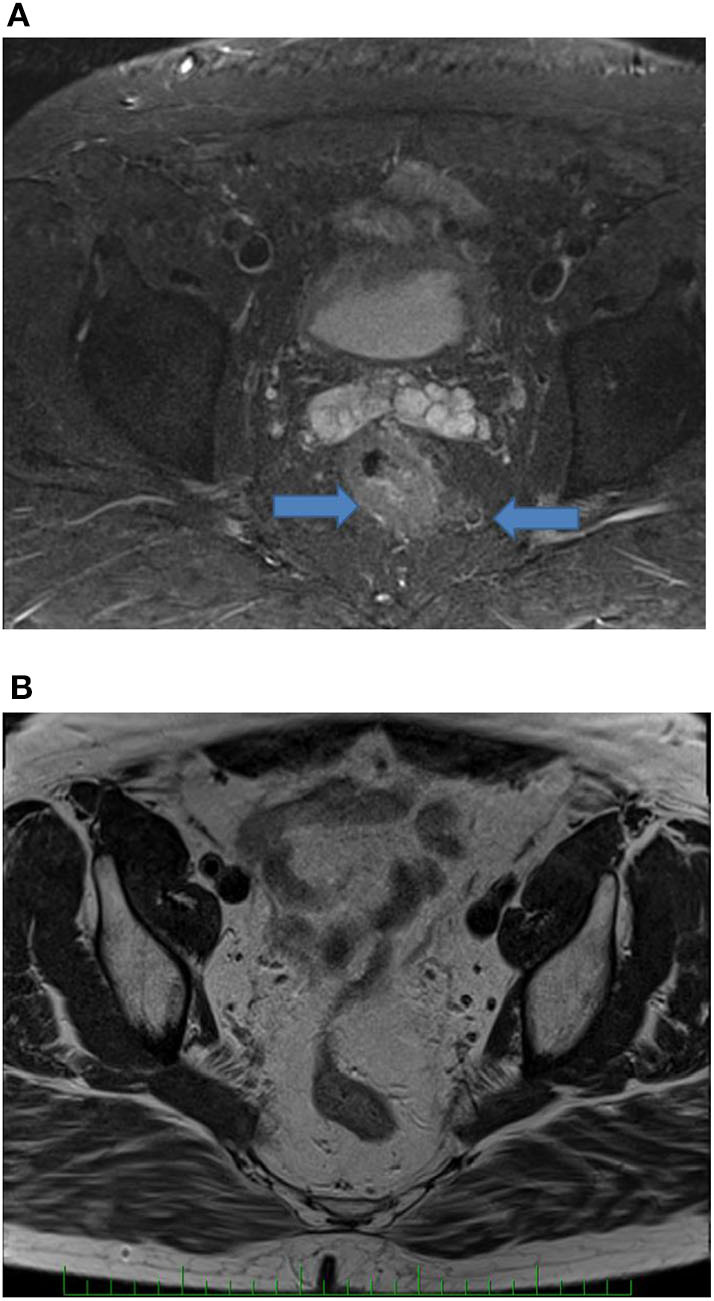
MRI of the rectal tumor (initially T3, N1) before (A) and after (B) treatment. Arrows show tumor and enlarged lymph node. After treatment, no tumor and lymph nodes are presented (complete clinical tumor response).
